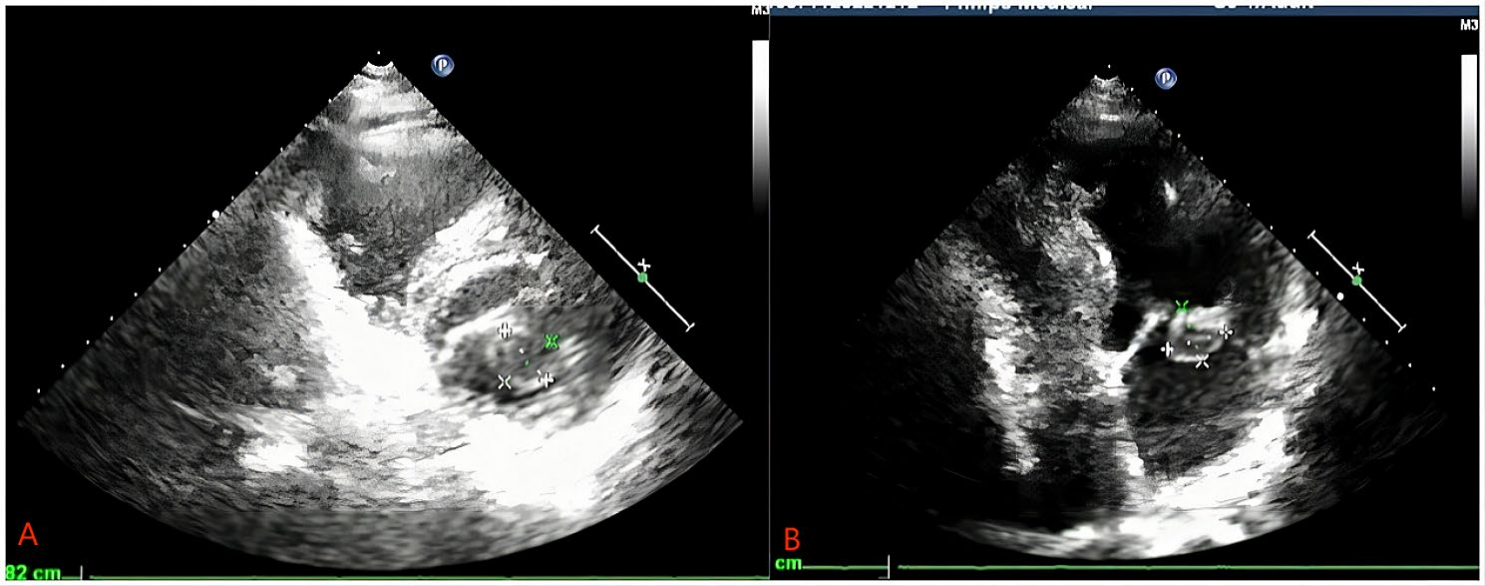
Echocardiography shows a cystic mass attached to the posterior leaflet of the mitral valve containing liquid (A,B)
